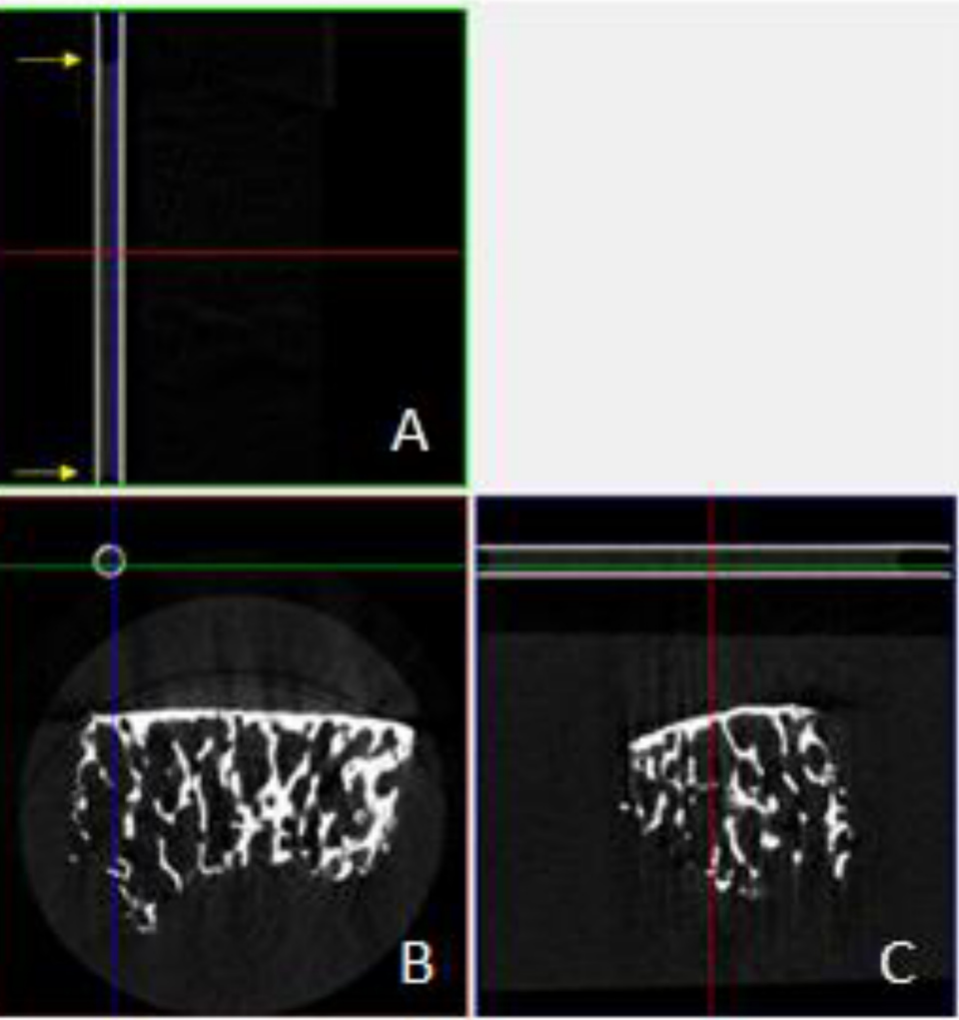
An example of aligning a micro-computed tomography scan of the K-F1 sample with the capillary wall (two parallel white lines), shown in the coronal (A), transaxial (B), and sagittal (C) planes. Blue and green lines, corresponding to x- and y-axis, are parallel to the capillary wall at top left and bottom right images, respectively. Yellow arrows point to the water level in the capillary.
